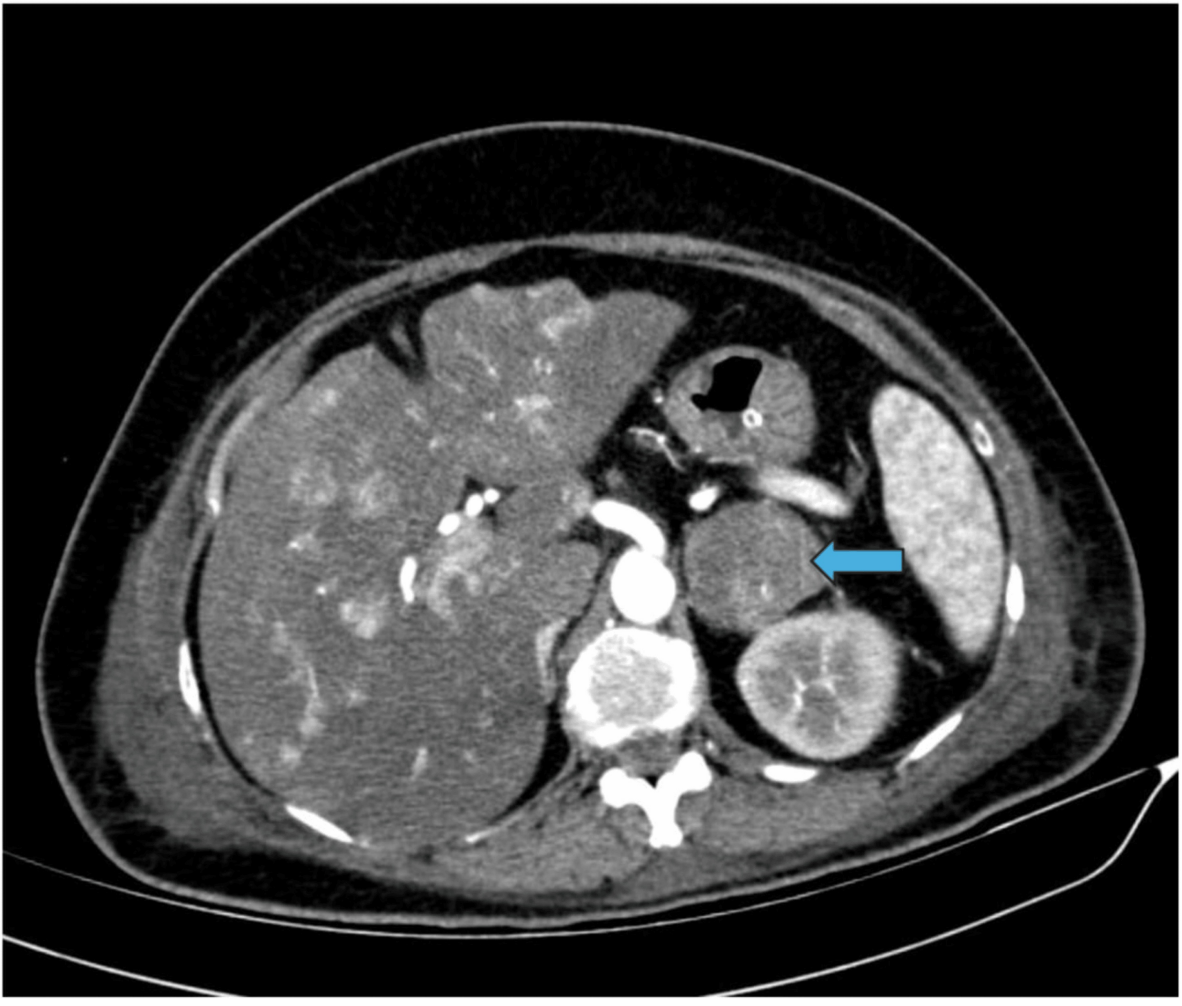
Contrast-enhanced CT abdomen showing a heterogenous enhancing mass in the left suprarenal region (blue arrow)
